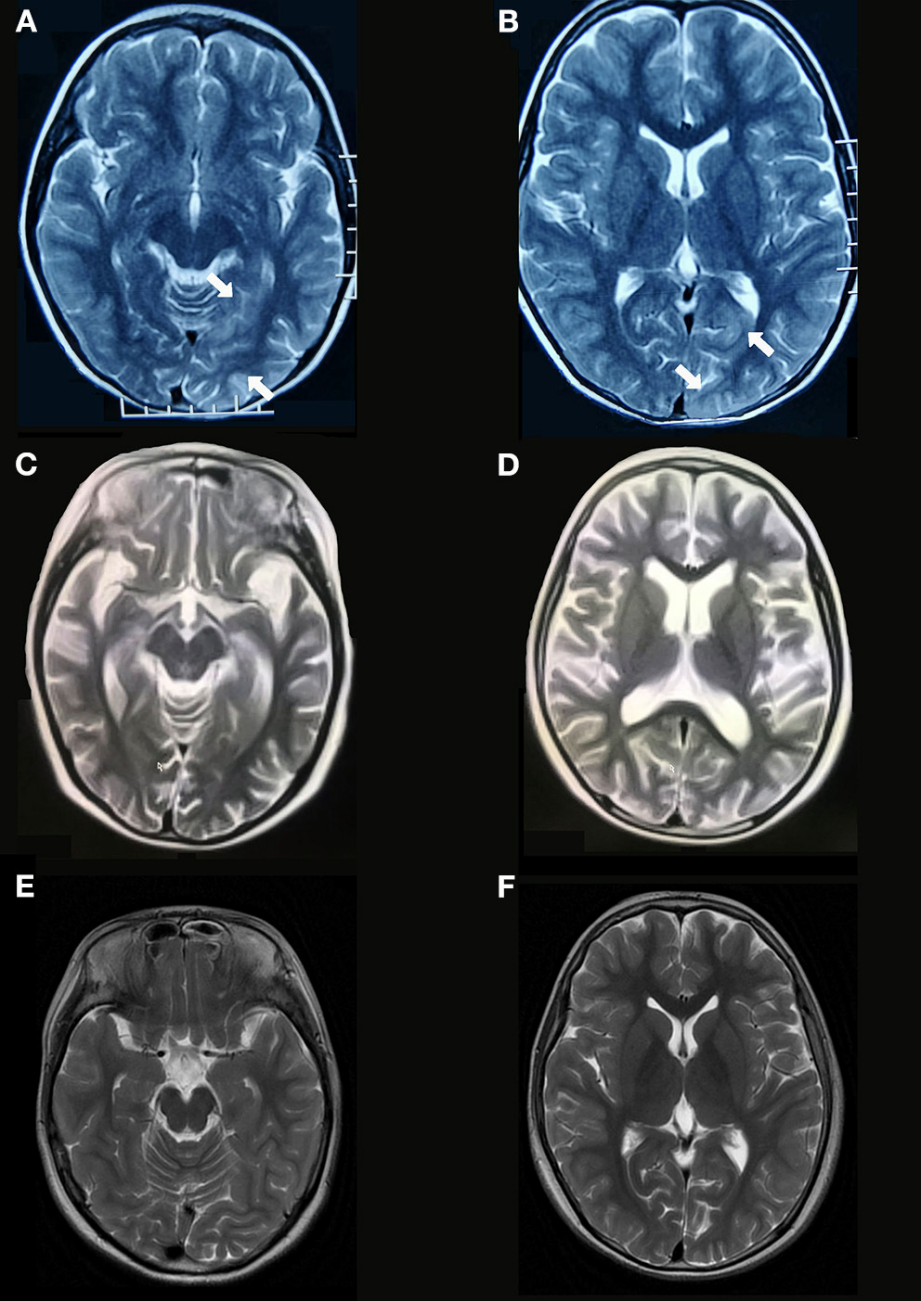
Brain MRI findings of anti-NMDAR encephalitis. Brain MRI obtained 5 days after symptom onset (patient 1, Table 1) shows increased T2 signal abnormalities involving in left temporal and occipital lobes (A,B). Diffuse brain atrophy (C,D) are shown on T2 sequences of brain MRI obtained 14 months after symptom onset (before rituximab). Brain MRI at the last follow-up shows normal (E,F).
